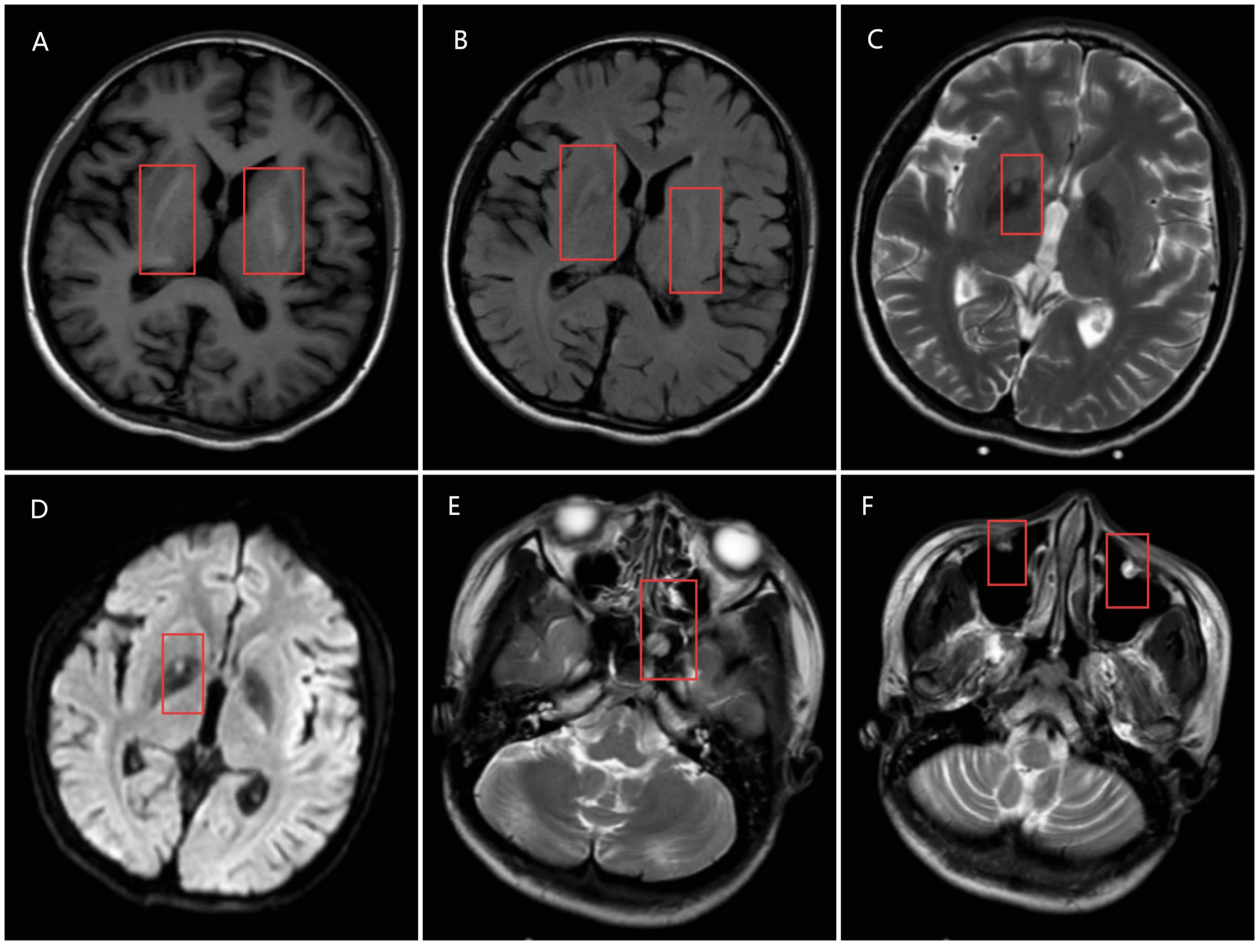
Head MRI. (A) T1-weighted image showing hyperintense lesions in the bilateral basal ganglia and thalamus (red mark). (B) T2-FLAIR image showing hyperintense lesions in the bilateral basal ganglia and thalamus (red mark). (C) Dot-like abnormal signal in the right putamen on T2-weighted images (red mark). (D) ADC map showing restricted diffusion in the right putamen (red mark). (E) Rounded hyperintense focus in the sphenoid sinus on T2-weighted images (red mark). (F) Hyperintense focus in the bilateral maxillary sinuses on T2-weighted images (red mark).
